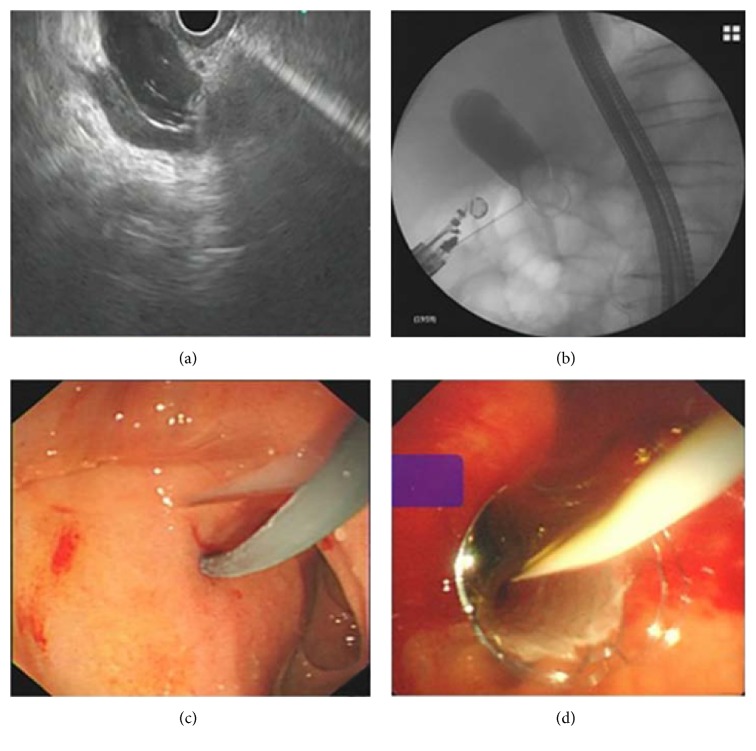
Endoscopic ultrasound-guided choledochoduodenostomy. Under real-time EUS guidance, the dilated extrahepatic bile duct was punctured and a cholangiogram was obtained (a and b). After biliary-enteric fistula dilation (c), a fully covered self-expandable metal stent was deployed (d).
